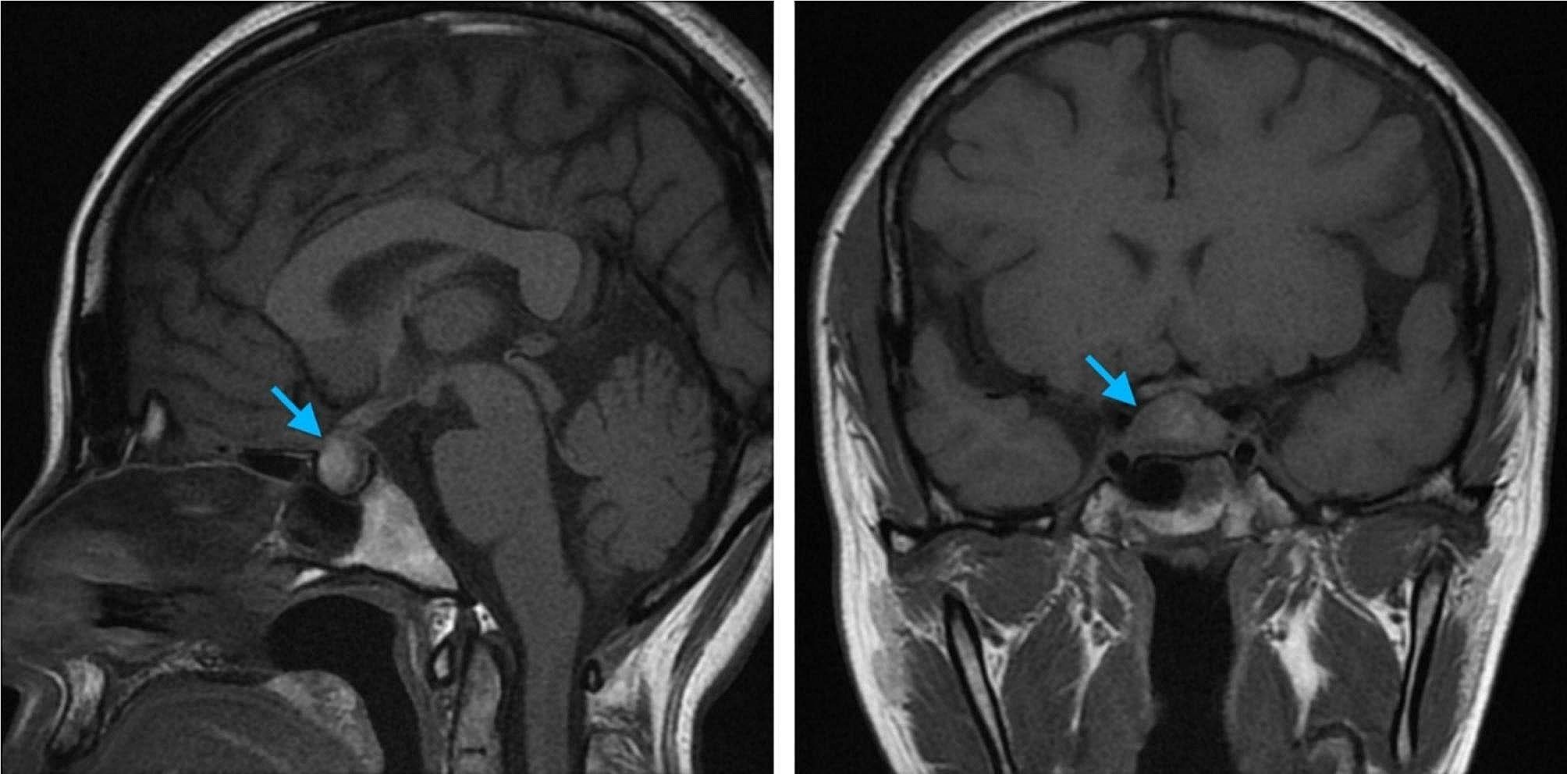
Blue arrow points to the inflamed pituitary gland, pre-op MRI. (A) Sagittal view of head MRI T1 without gadolinium contrast. Loss of posterior pituitary signal is present. (B) Coronal view of head MRI T1 without gadolinium contrast
